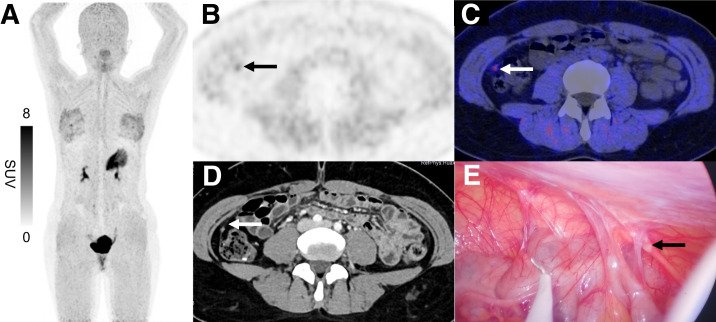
^68^Ga-FAPI-04 PET/CT images of 24-y-old woman with poorly differentiated gastric adenocarcinoma and peritoneal carcinomatosis. (A) Maximum-intensity-projection image showed no conspicuous abnormal tracer uptake in abdomen apart from primary tumor. However, axial PET (B) and PET/CT fusion (C) images revealed subtle focus in right paracolic gutter (arrows; SUV_max_, 1.71). (D) Corresponding contrast-enhanced CT demonstrated enhancing nodule that was initially missed. (E) Subsequent preoperative staging laparoscopy confirmed peritoneal implant at corresponding site (arrow).
